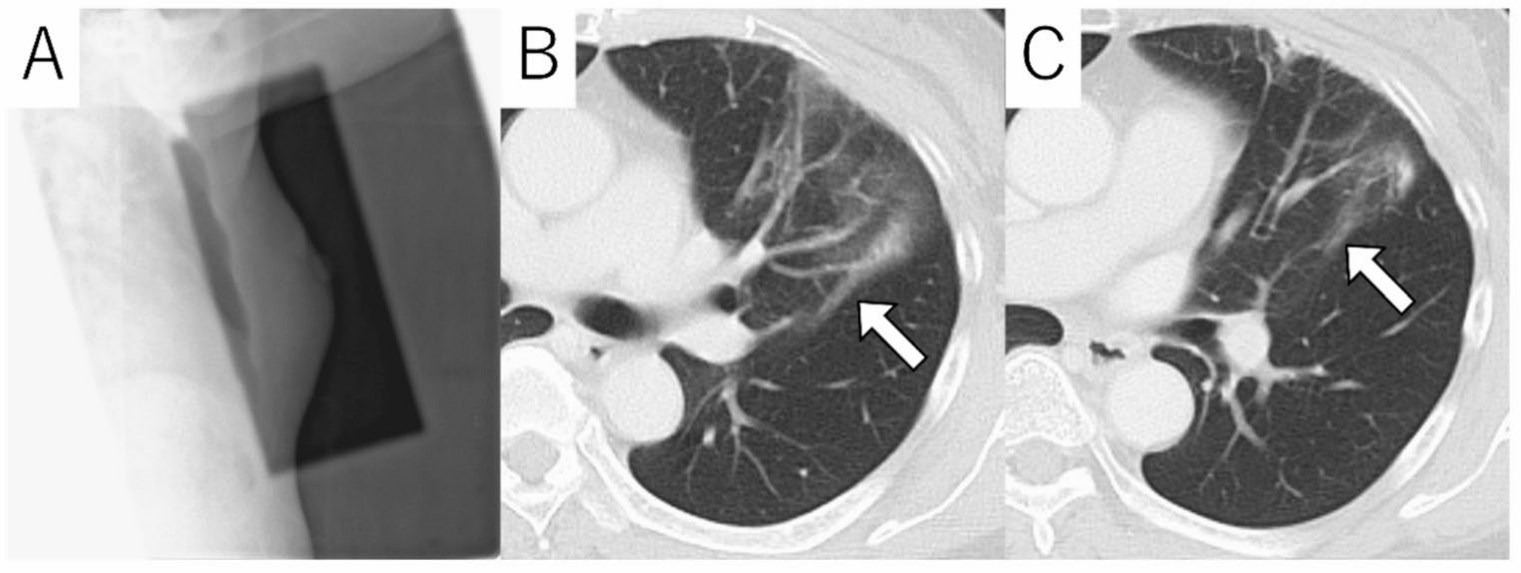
Radiation-induced organizing pneumonia (OP) in a 61-year-old with breast cancer. The patient underwent radiation therapy after breast-conserving surgery. Portal radiograph shows a tangential irradiated field (A). CT images show ground-glass opacities and consolidations extending beyond the irradiated field (arrows) (B, C)
